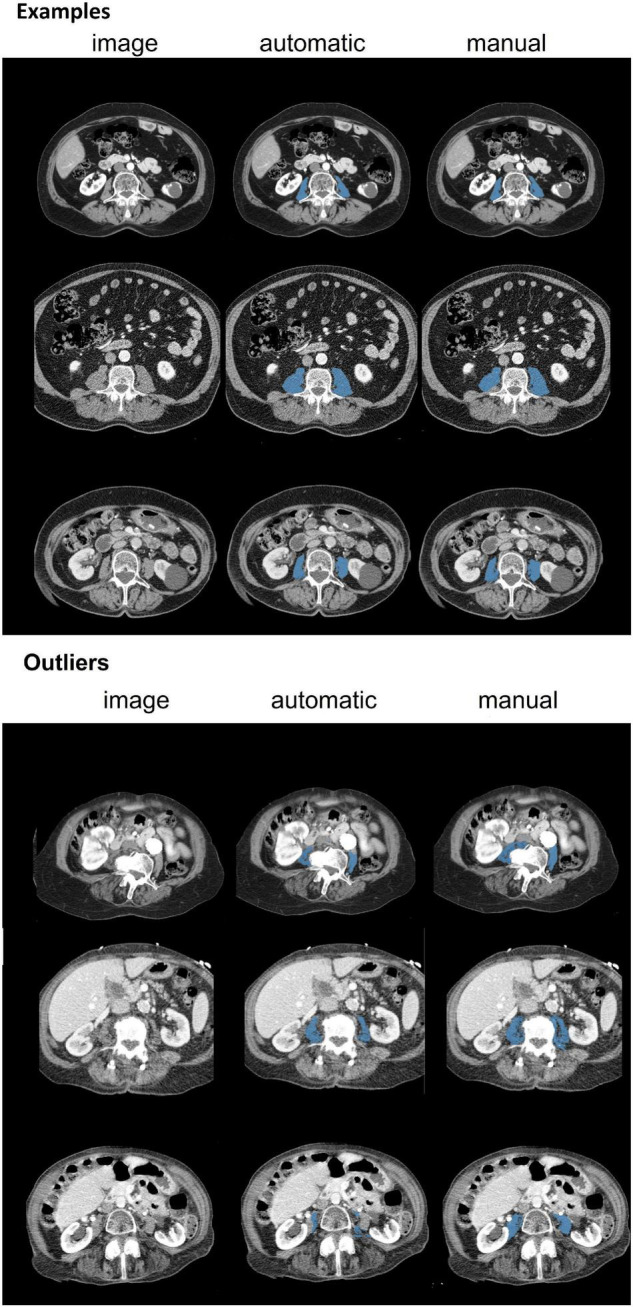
Examples of three randomly selected CT scans and three largest outliers for 6 different patients. Dice indices for these examples were 0.92 (top row), 0.90 (middle row) and 0.93 (bottom row). Dice indices for outliers were 0.74 (top row), 0.75 (middle row) and 0.36 (bottom row). The outlier shown in the top row contains an artifact, the middle row shows an overexposed vertebra and the bottom row shows an uncommon distribution of connective tissue, organs and muscle area and is incorrectly segmented by the automatic software.
